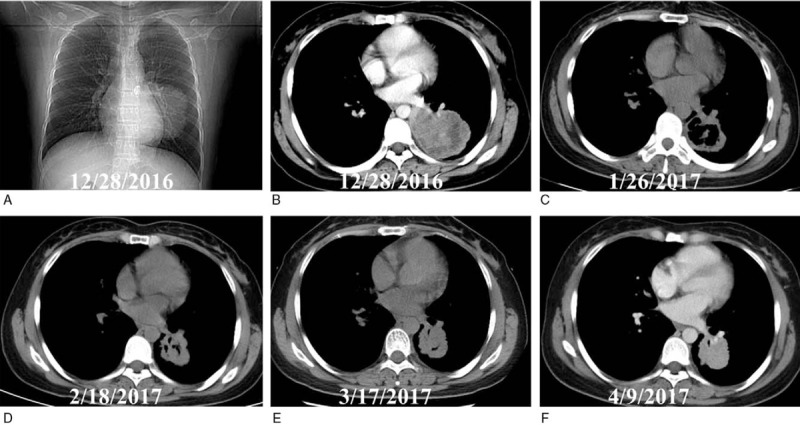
Chest x-ray and CT images of the pulmonary tumor during the induction treatment. A, X-ray on admission showed a bulky mass located in the left lower lobe. B, CT showed that the mass was 70 mm × 60 mm in size. C, One month after oral apatinib plus S-1, the tumor indicated partial remission (PR) measuring 43 mm × 54 mm with a necrotic cavity. D, Two months after induction therapy, the tumor showed stable disease (SD) measuring 44 mm × 37 mm. E, The lesion was 41 mm × 40 mm in size 3 months after treatment and SD was indicated. F, Four months after, the tumor was slightly enlarged measuring about 41 mm × 42 mm before surgery. CT = computed tomography.
